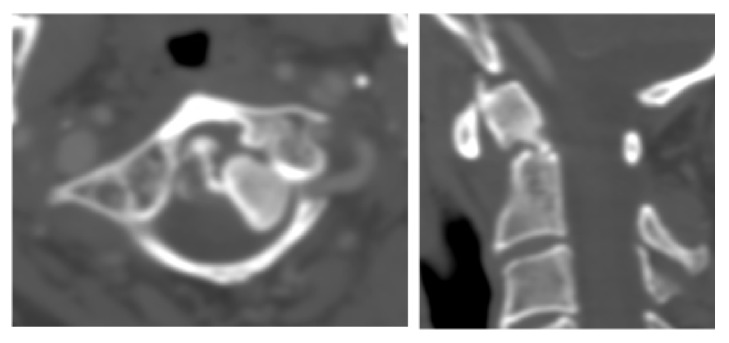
Transversal and sagittal CT imaging of C1 and C2, showing luxation of the left atlantoaxial facet joint (type II according to Fielding [9]) combined with a fracture of the odontoid process (type II according to Anderson and D‘Alonzo).
